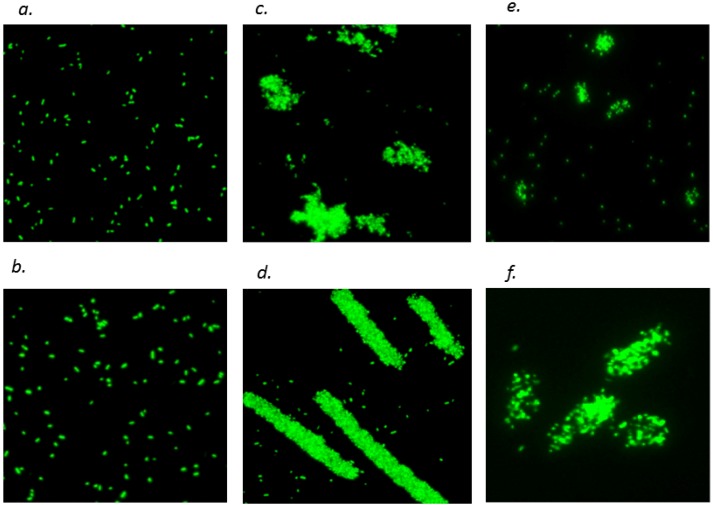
Aggregation of *Escherichia coli* (strain 25922) cell in the presence of condensed tannins (CT) of purple prairie clover (PPC; *Dalea purpurea* Vent.) and sainfoin (SF; *Onobrychis viciifolia*). *a* and *b*, bacterial suspensions prepared from culture pre-incubated with 0 and 10 µg/mL of PPC CT for 10 h; *c* and *d*, bacterial suspensions added with 50 and 200 µg/mL of PPC CT; *e* and *f*, bacterial suspensions added with 50 and 200 µg/mL of SF CT.
